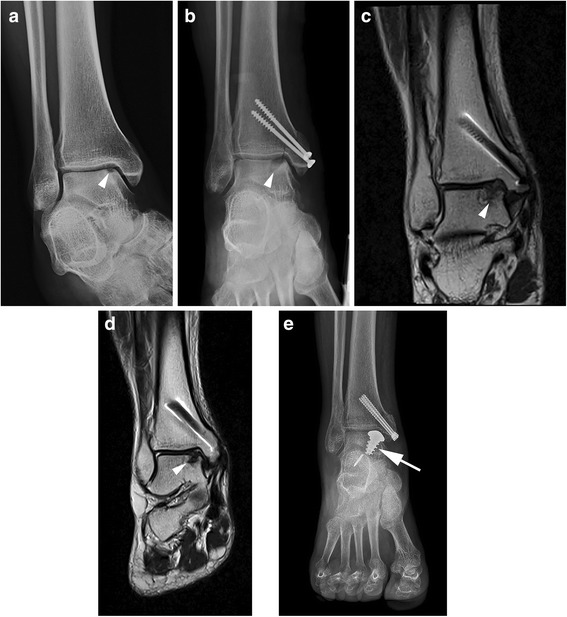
Fifty-three year old woman with OLT repaired using AMIC technique with MaioRegen. Preoperative (a) and postoperative (b) conventional radiographs show a medial talar dome osteochondral lesion (*headarrows*). Coronal T2-weighted turbo spin-echo images at 12 months (c) and 24 months (d) show complete filling of the osteochondral defect of the medial talar dome (*headarrows*). Conventional radiograph performed after re-intervention (e) with implantation of talar dome HemiCAP® (*arrow*)
